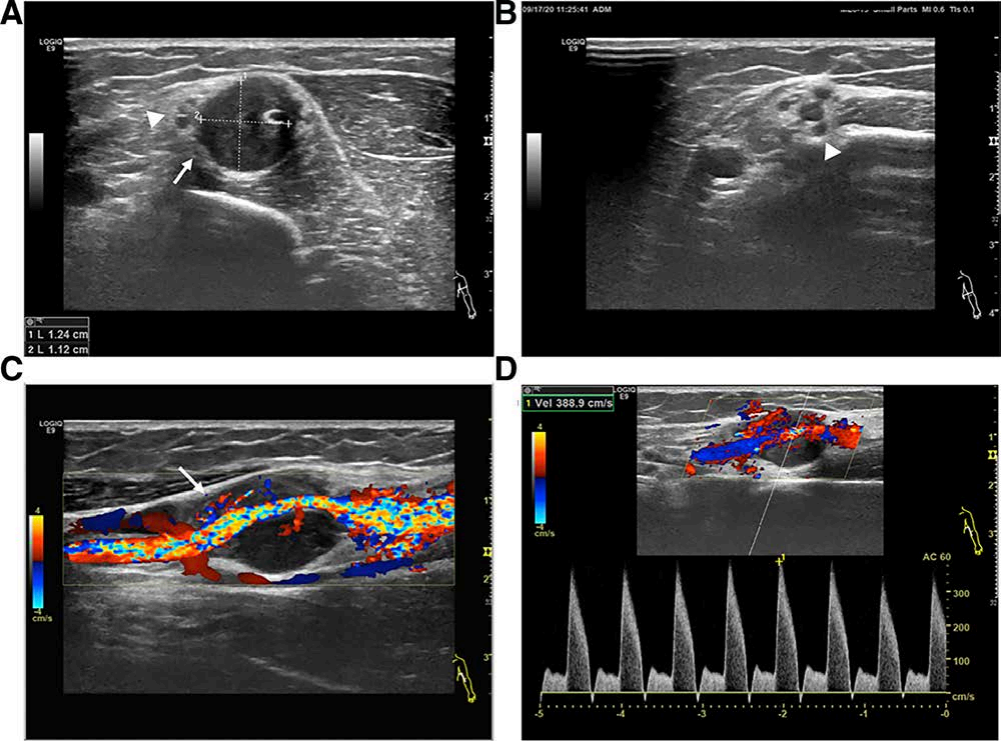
Ultrasonography of the left brachial artery (BA). (A, B) A hypoechoic mass in the middle layer of the left BA (12 × 11mm), with regular morphology and clear boundaries (white arrow). The peripheral median nerve is compressed with edema (white arrowheads). (C) Longitudinal section examination reveals a hypoechoic mass between the outer wall and the lumen of the vessel with abundant blood flow running through, and the lumen is narrowed and distorted (white arrow). (D) Doppler ultrasound detects severe stenosis of the BA (the peak speed is 389cm/s).
